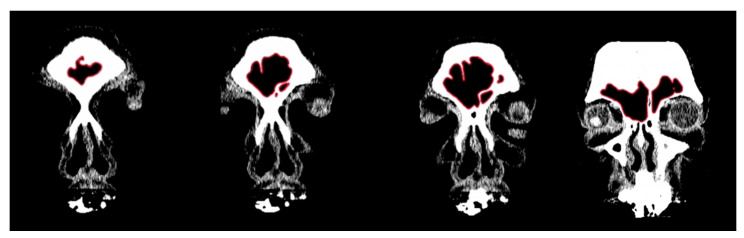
Example of CT image slices from a study individual with the frontal sinuses traced in red in Adobe Photoshop.
